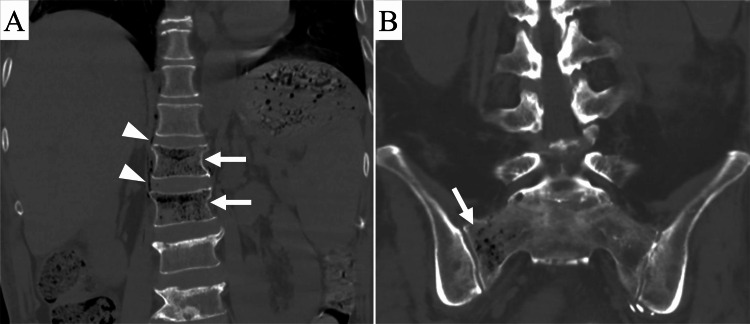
Computed tomography (CT) images of the lumbosacral spine obtained 112 days after liver transplantation. (A) Coronal CT image of the lumbar spine shows clusters of small intraosseous gas (arrows) in the first and second lumbar vertebral bodies, extraosseous gas (arrowheads) around the vertebral bodies, and compression fractures of the first and second lumbar vertebral bodies. (B) Coronal CT image of the sacrum shows clusters of small intraosseous gas (arrow) in the right sacral ala.
